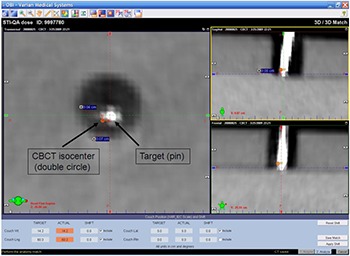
Difference measurement on CBCT workstation. The CBCT isocenter is shown as a double circle in axial, sagittal and coronal images on this workstation. The difference between the CBCT isocenter and the target was obtained using the measurement tool of the CBCT workstation.
